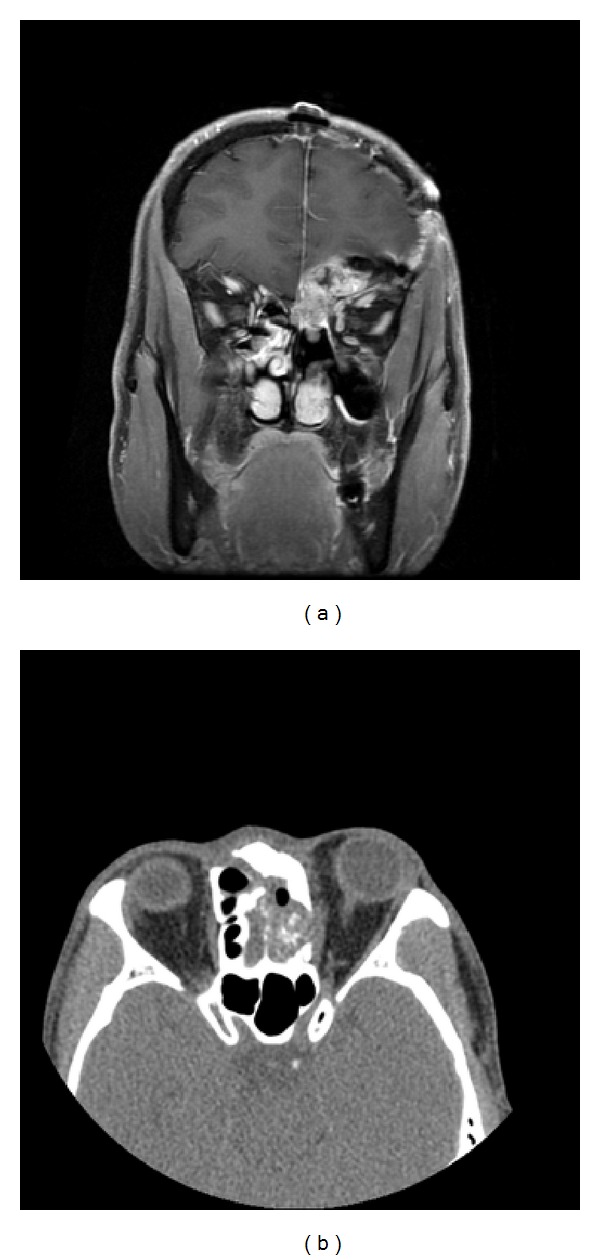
Sinus MRI (coronary view) and sinus CT scan (axial view) of sinonasal inverted papilloma with malignant transformation.
